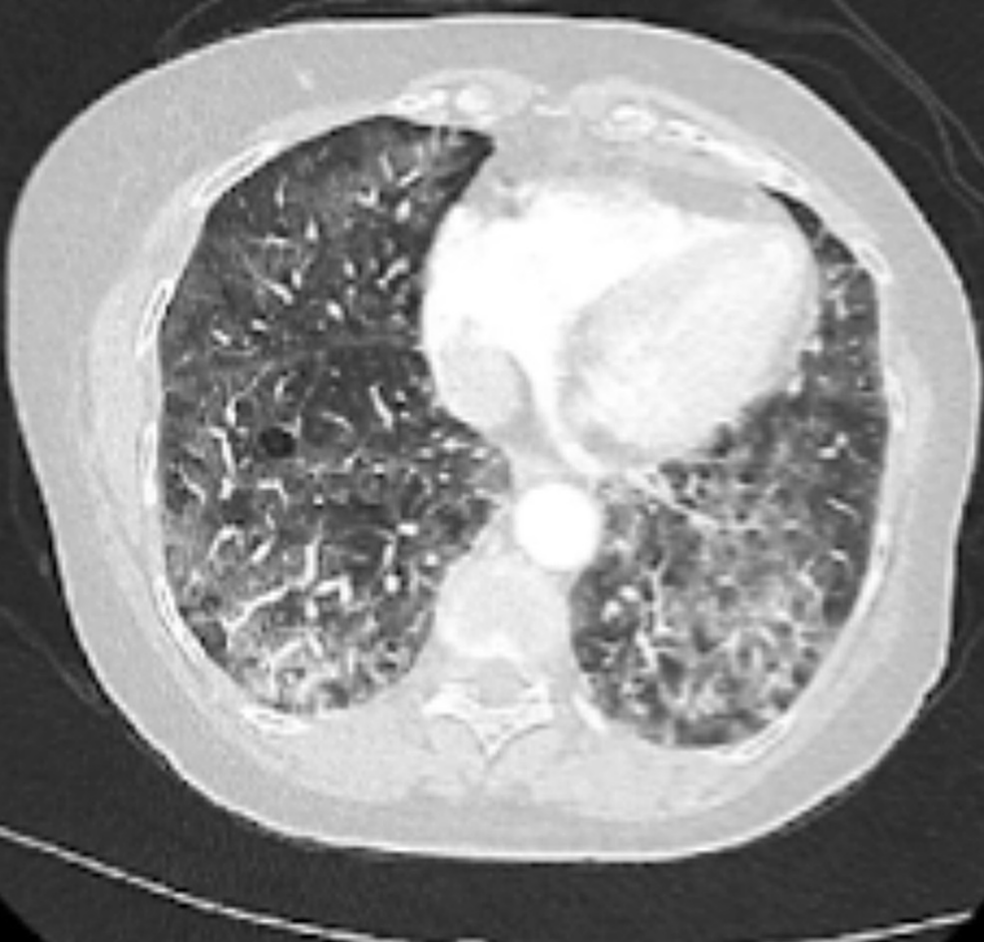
CT angiogram of the chest showing advanced centrilobular emphysema with nonspecific thickening of the pulmonary septa and associated ground-glass opacity predominating the mid to lower lung field.
